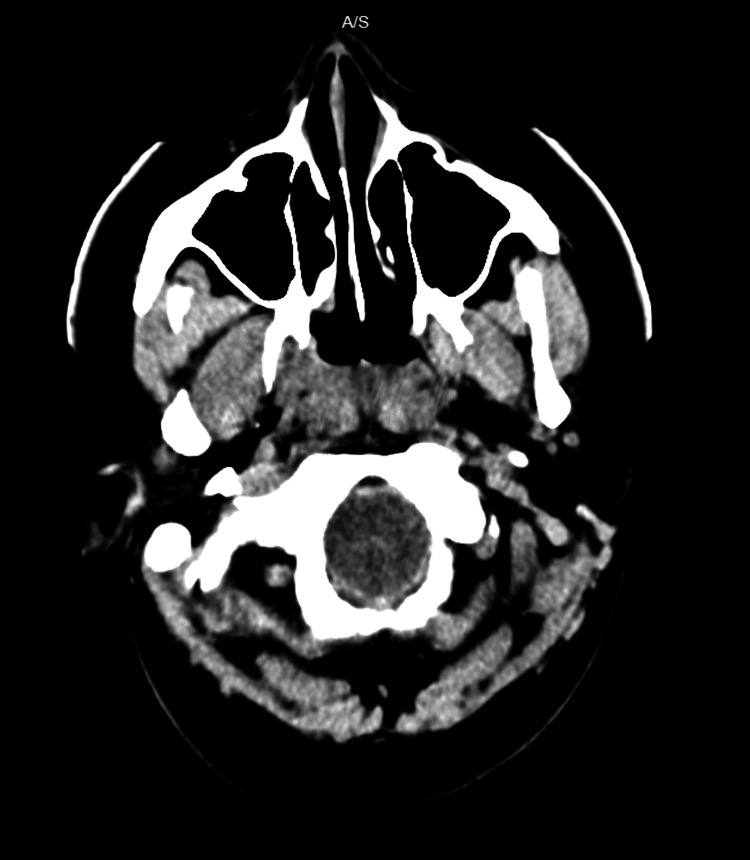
CT head inferior slice Crowding at the skull base is observed with effacement of the basal cisterns and apparent compression at the level of the foramen magnum, concerning for downward herniation.
